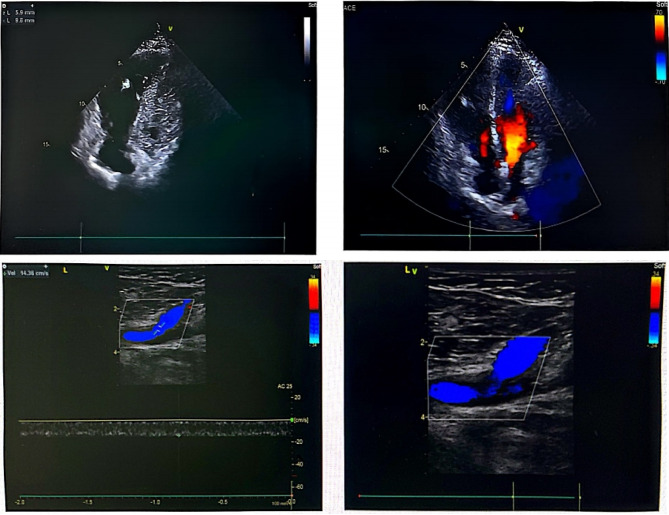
Imaging findings of this admission. UCG showed a 9.8 mm x 5.9 mm hyperechoic mass in the right ventricular cavity, irregular and oscillating with heartbeat. The internal diameters of the right and left pulmonary arteries were 15.3 mm and 13.7 mm, respectively, with an irregular hypoechoic mass proximal to the left pulmonary artery and irregular blood flow distally. CDFI showed thrombosis in the external iliac veins to the common iliac vein of the lower extremities bilaterally, with partial recanalization of the lumen and thrombosis of the distal cardiac appendages. Ultrasound showed thickening of the wall of the bladder, and there was no abnormality of the liver, gallbladder, pancreas, or spleen
